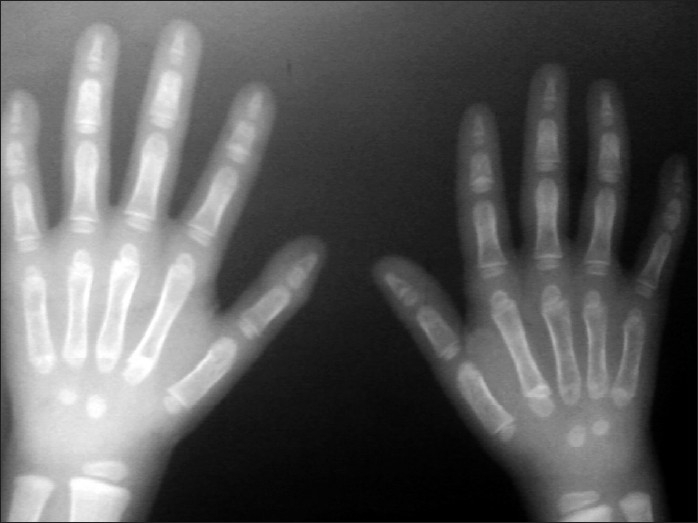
X-ray hand showing only two carpal bones
